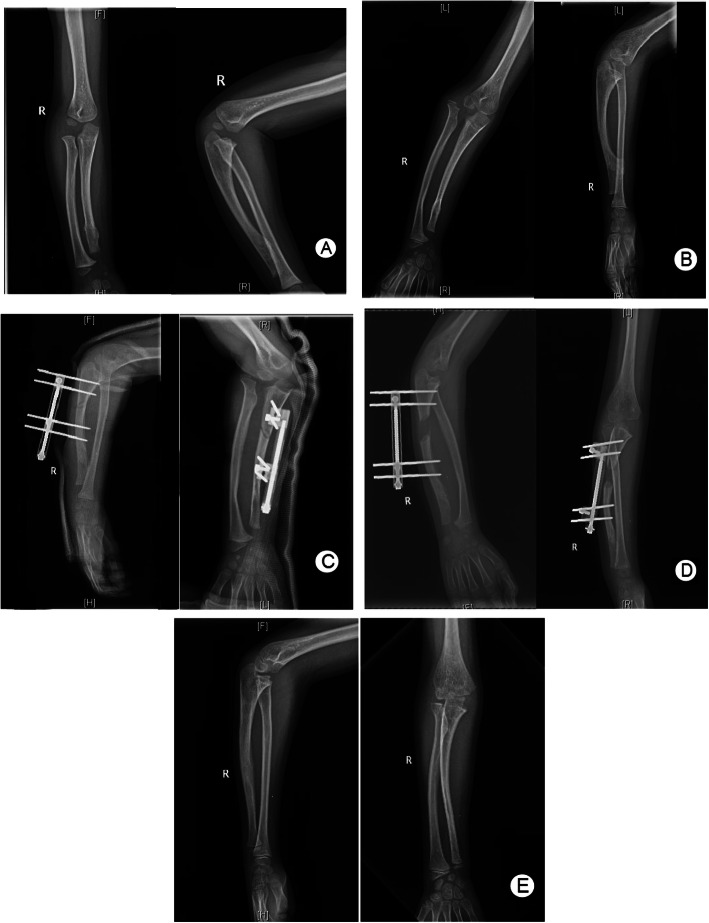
A girl of Masada type IIb forearm deformity: (a) 3.5-year-old, X-rays. Forearm deformity was not obvious, with good elbow function. (b) 6.5-year-old, preoperative X-rays. Ulnar shortening, ulnar deviation of wrist, radial head dislocation and cubitus varus were obvious, with flexion and pronation disorder of elbow. (c) Early postoperative X-rays. Osteochondroma resection and ulna osteotomy were performed, with the Monorail external fixator ready for ulnar lengthening. (d) 1 month after operation, 2.5cm were gained. (e) 9.5-year-old, X-rays of the latest follow-up. Spontaneous reduction of radial head was observed, and the distal ulna obtained a certain growth potential. The forearm deformity was corrected
